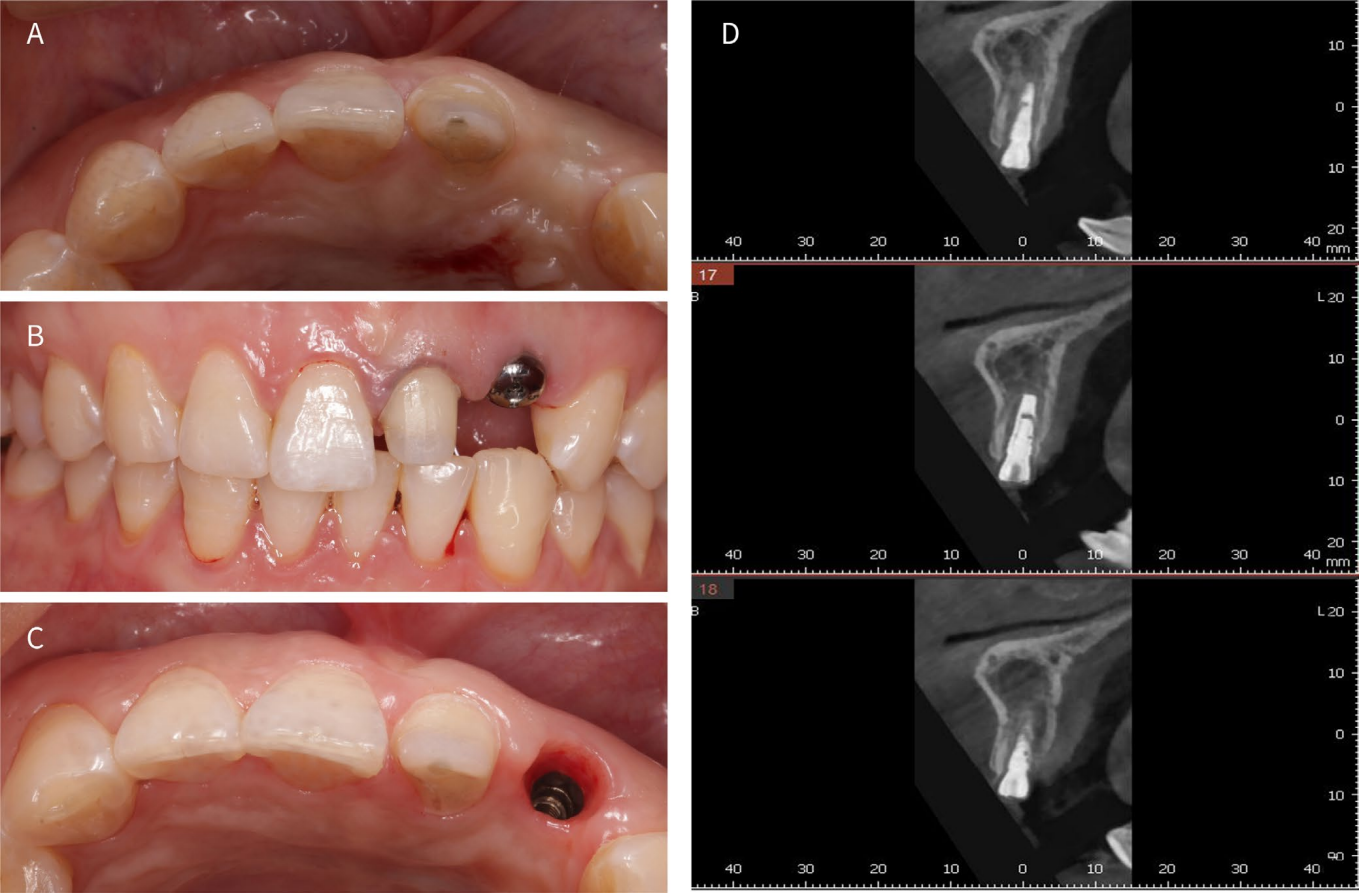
(A) Three‐month follow‐up healing assessment; (B) 1 month after healing abutment placement; (C) Soft‐tissue emergence profile after 1 month of healing abutment placement; (D) No hard‐tissue resorption was detected from the cone beam computed tomography image.
